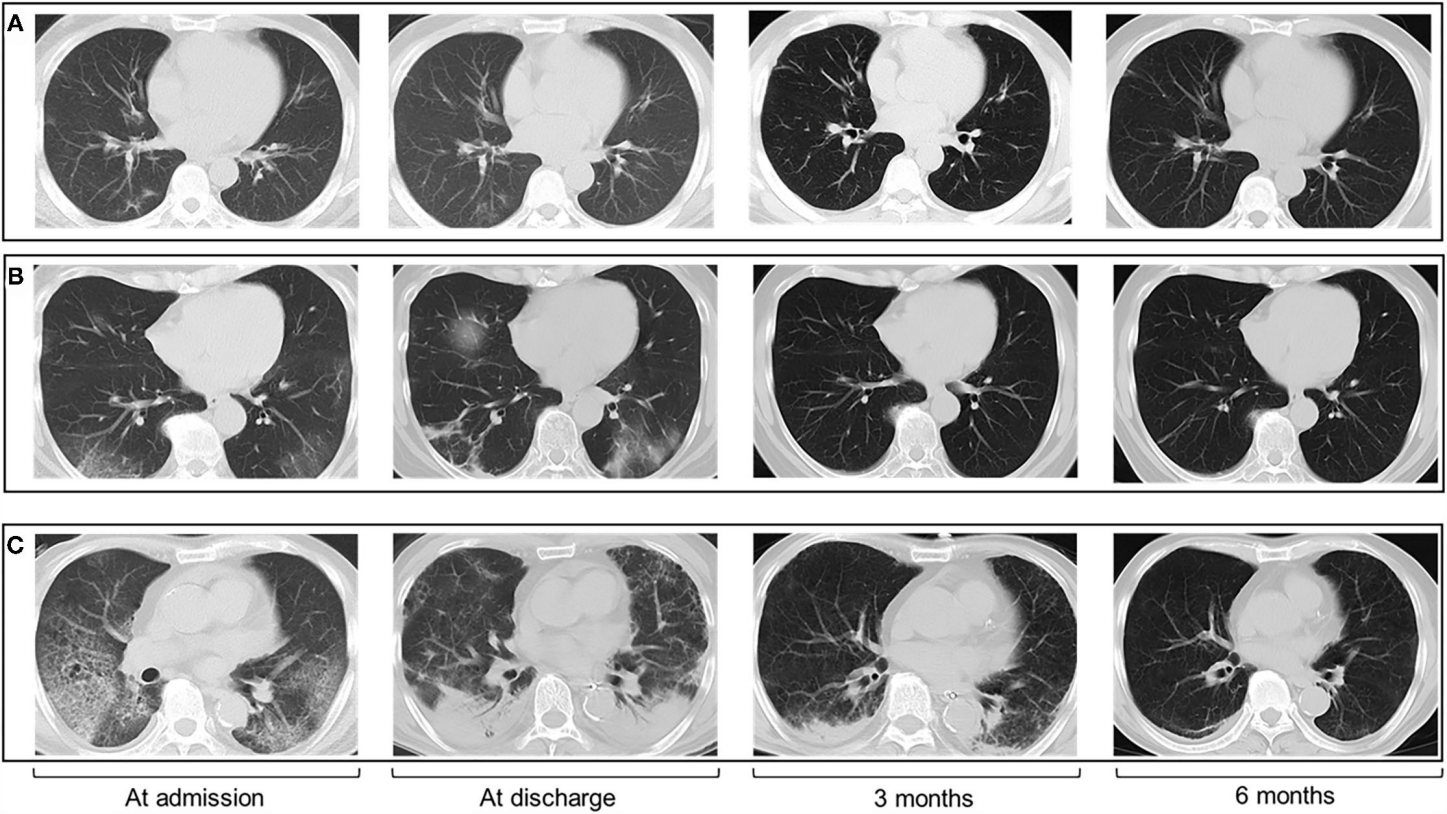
Chest CT images of patients with COVID-19 at four timepoints (admission, discharge, 3 months, and 6 months follow-up). The CT scans show GGO with bilateral lung involvement at admission and patients with greater disease severity had more residual CT abnormalities. (A) CT scans in a 48-year-old man with moderate illness. (B) CT scans in a 55-year-old woman with severe illness. (C) CT scans in a 67-year-old man with critical illness.
